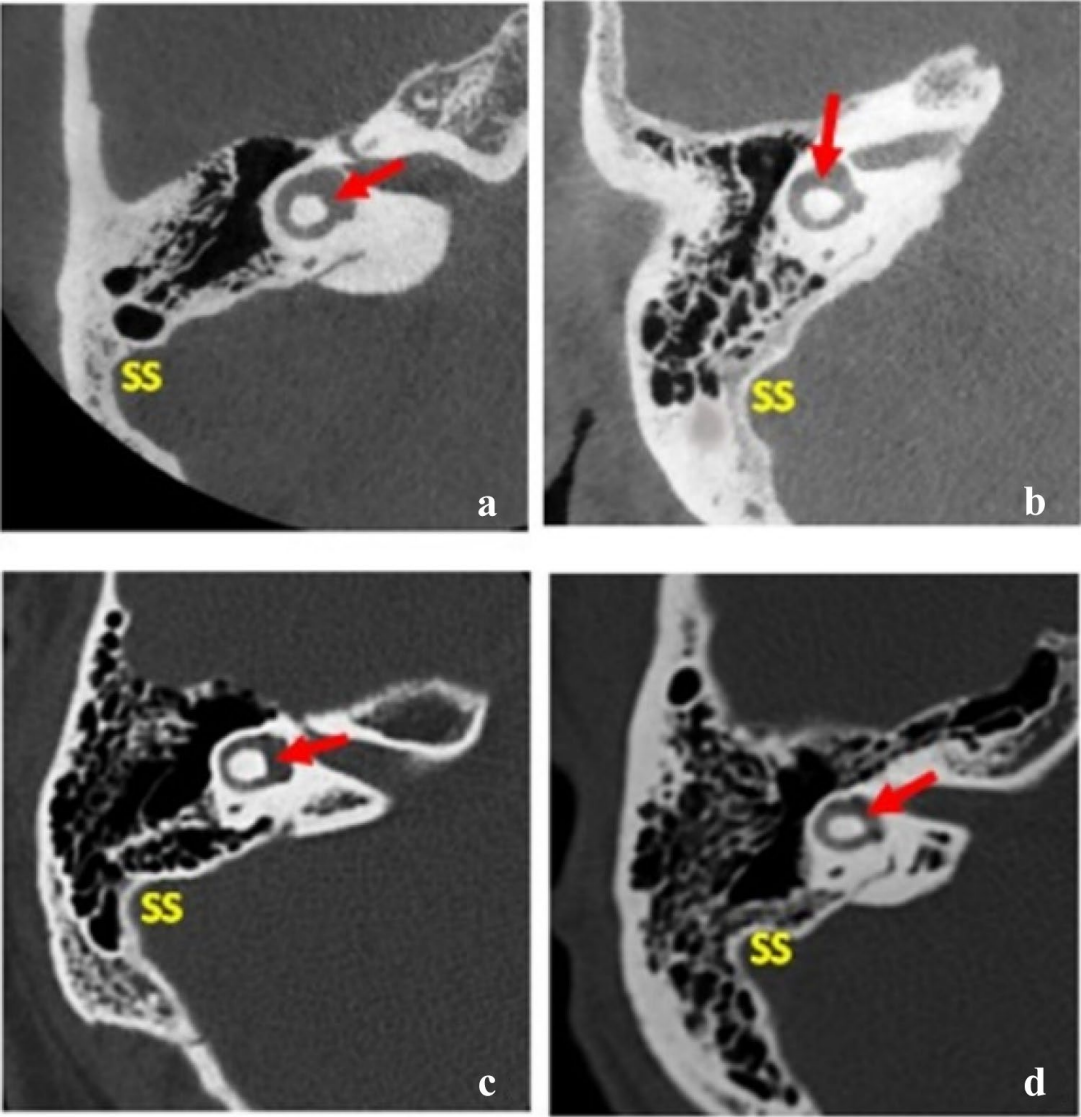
A representation of axial CT images taken at the level of the LSCC (red arrow) used in the questionnaire showing different degrees of pneumatization after dividing the lateral convex or semicircular-shaped line/border of the sigmoid sinus into three equal segments using two imaginary dots. a hypo-pneumatization; b moderate pneumatization; c good pneumatization; d) hyper-pneumatization. *SS* sigmoid sinus (Color figure online)
